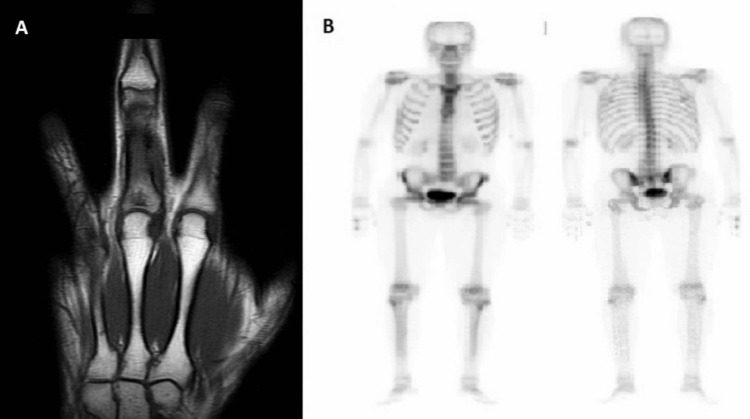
(A) Coronal T1-weighted magnetic resonance imaging of the right hand (2016). (B) Bone scintigraphy (2016).
